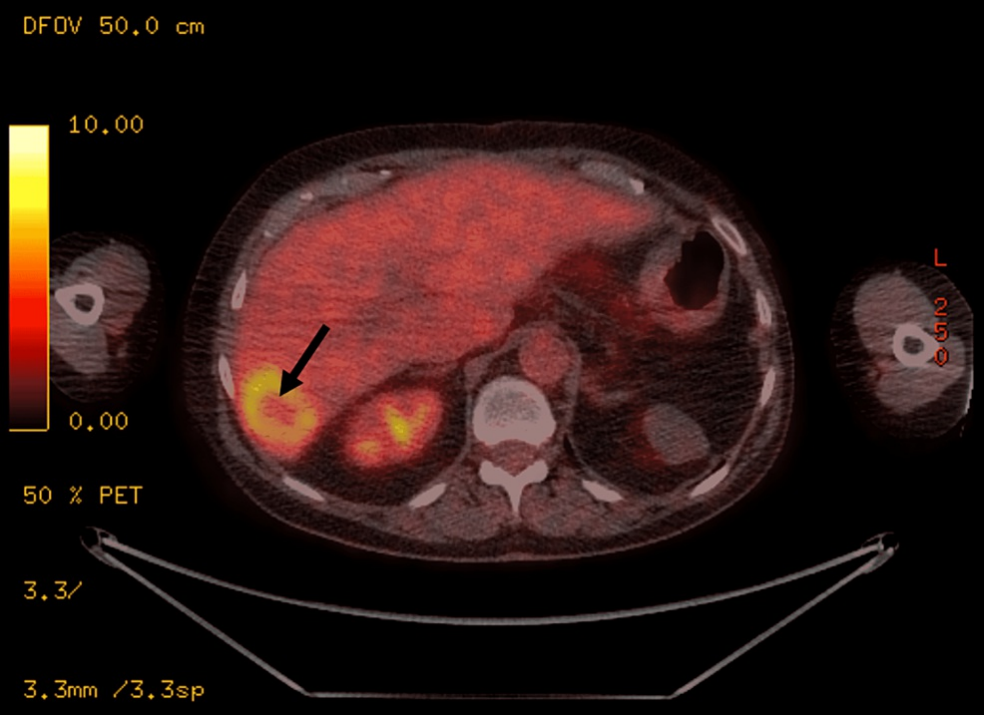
Outpatient PET-CT (post-SIRT). PET-CT three months post-SIRT treatment showing a reduction in the size of the segment 6 metastatic deposit from 5.5 cm to 3.5 cm. There was a reduction in the maximal FDG avidity to 8.4, with central necrosis present (black arrow). PET-CT: positron emission tomography-computed tomography; SIRT: selective internal radiation therapy
